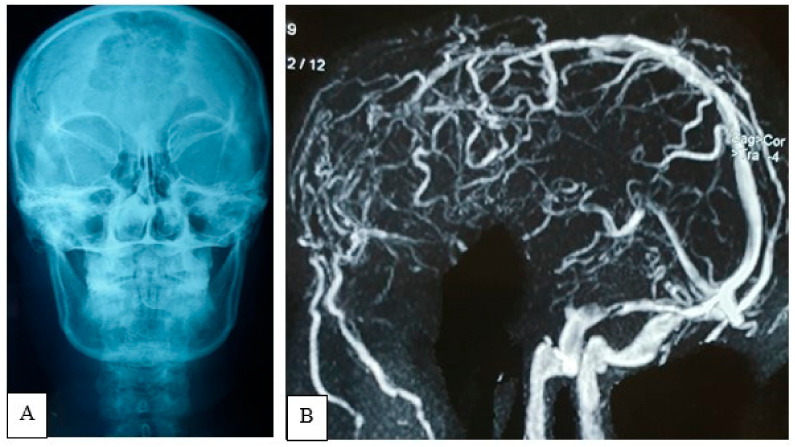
Plain X-ray of the skull, AP view (A) showing expansile lytic lesion with internal septation is noted within frontal bone. MRV (B) oblique view demonstrates anterior third of the SSS obliterated with multiple aberrant collaterals.
